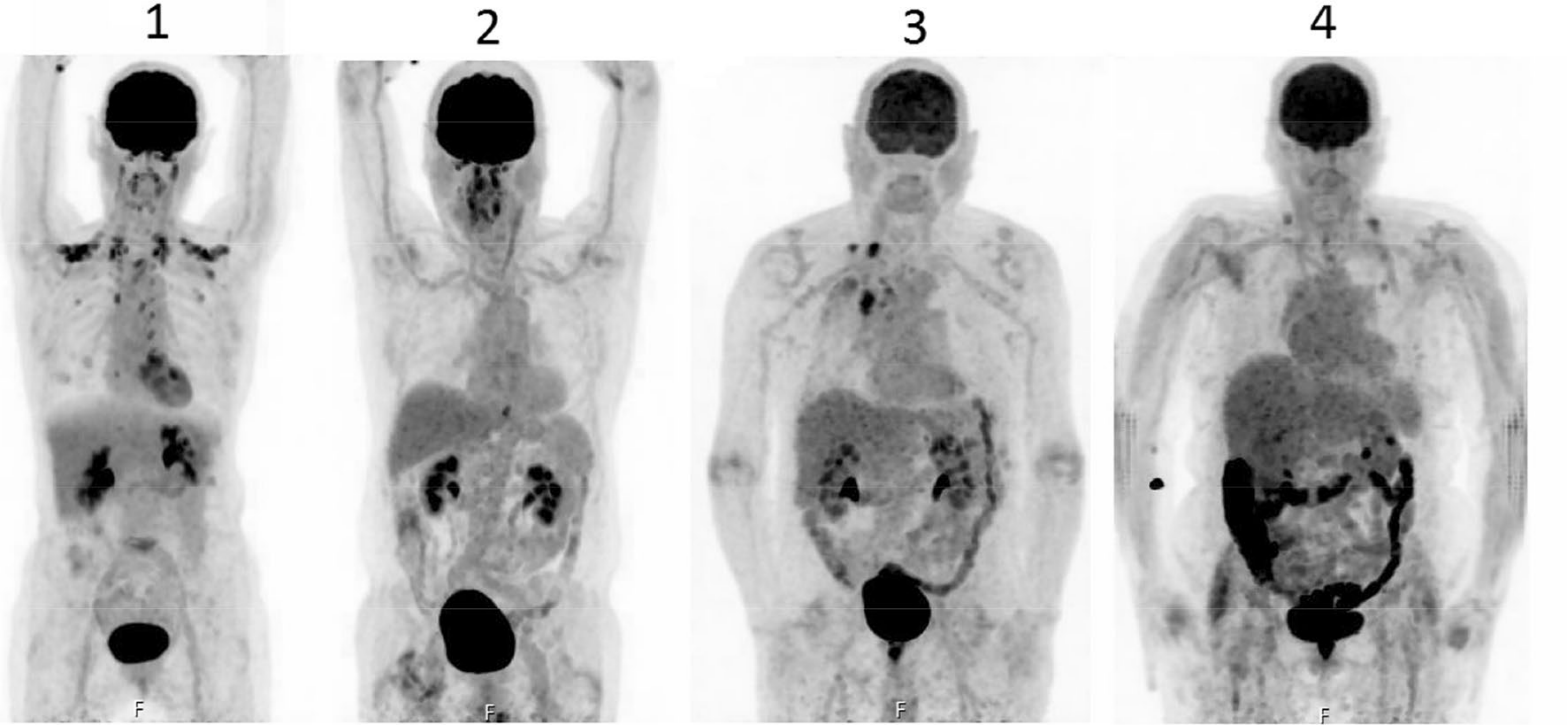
PET/CT images of four patients with different grades of FDG uptake in the colon, from left to right, corresponding to the four-point scale method of Gontier et al. [2]
